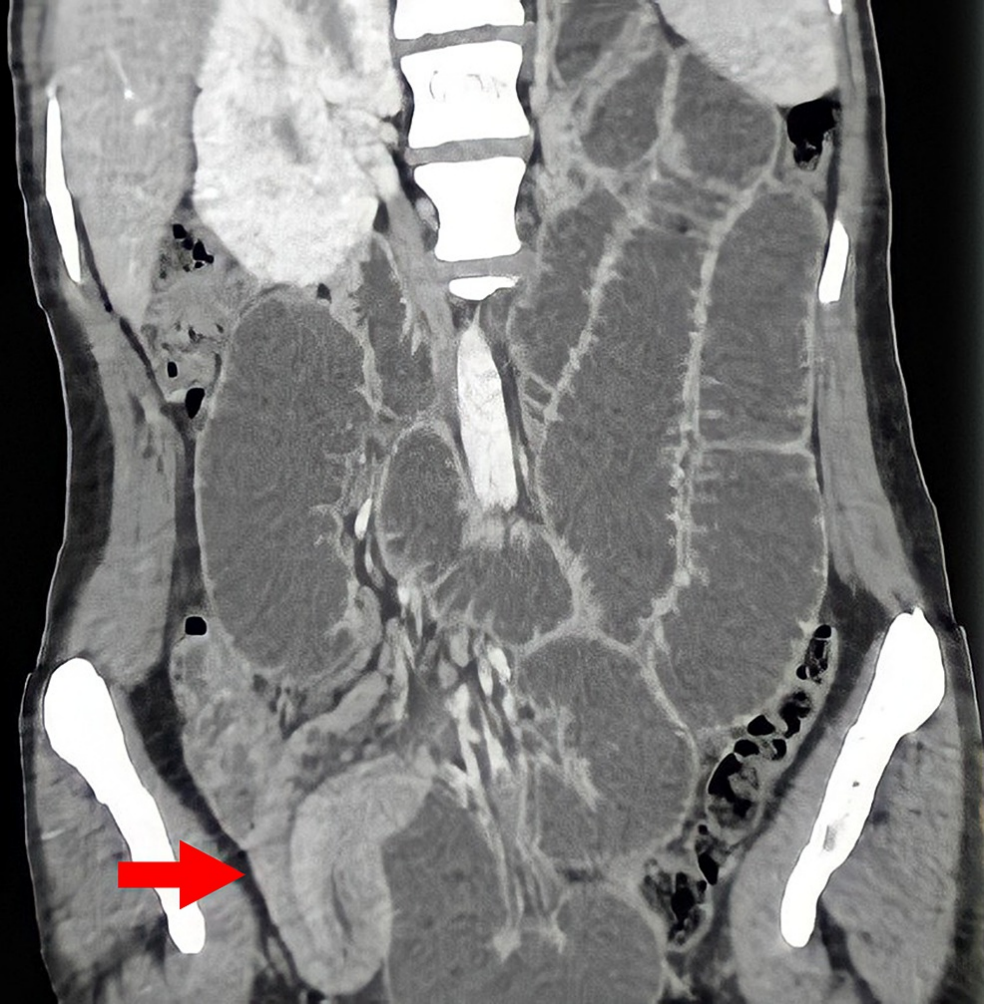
CT scan showing ileo-ileal invagination producing a sandwich image (red arrow)
